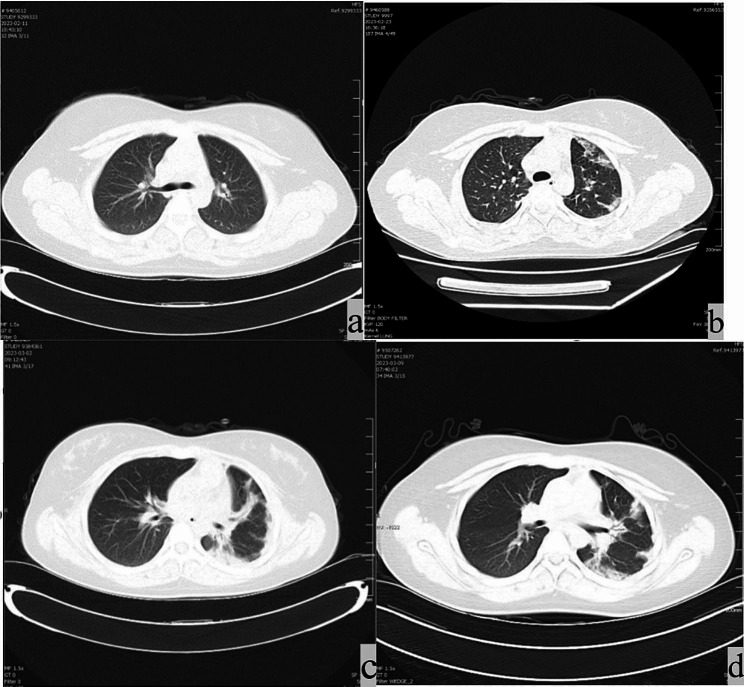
Chest CT results: a Case B on the second day of admission, revealing no obvious abnormalities. b Case B on day 13 of admission, revealing the interstitial exudation in both lungs, with the most pronounced effect for the left lung. c Case B on day 20 of admission, revealing the infection of both lungs with bronchial traction, dilation of the left lung, and bilateral pleural thickening. d Case B on day 27 of admission, revealing interstitial pneumonia in both lungs, slightly larger exudative lesions than in the previous film, with no apparent change in lesion range and a limited amount of pleural effusion on the left side; 2. Signs of anemia
